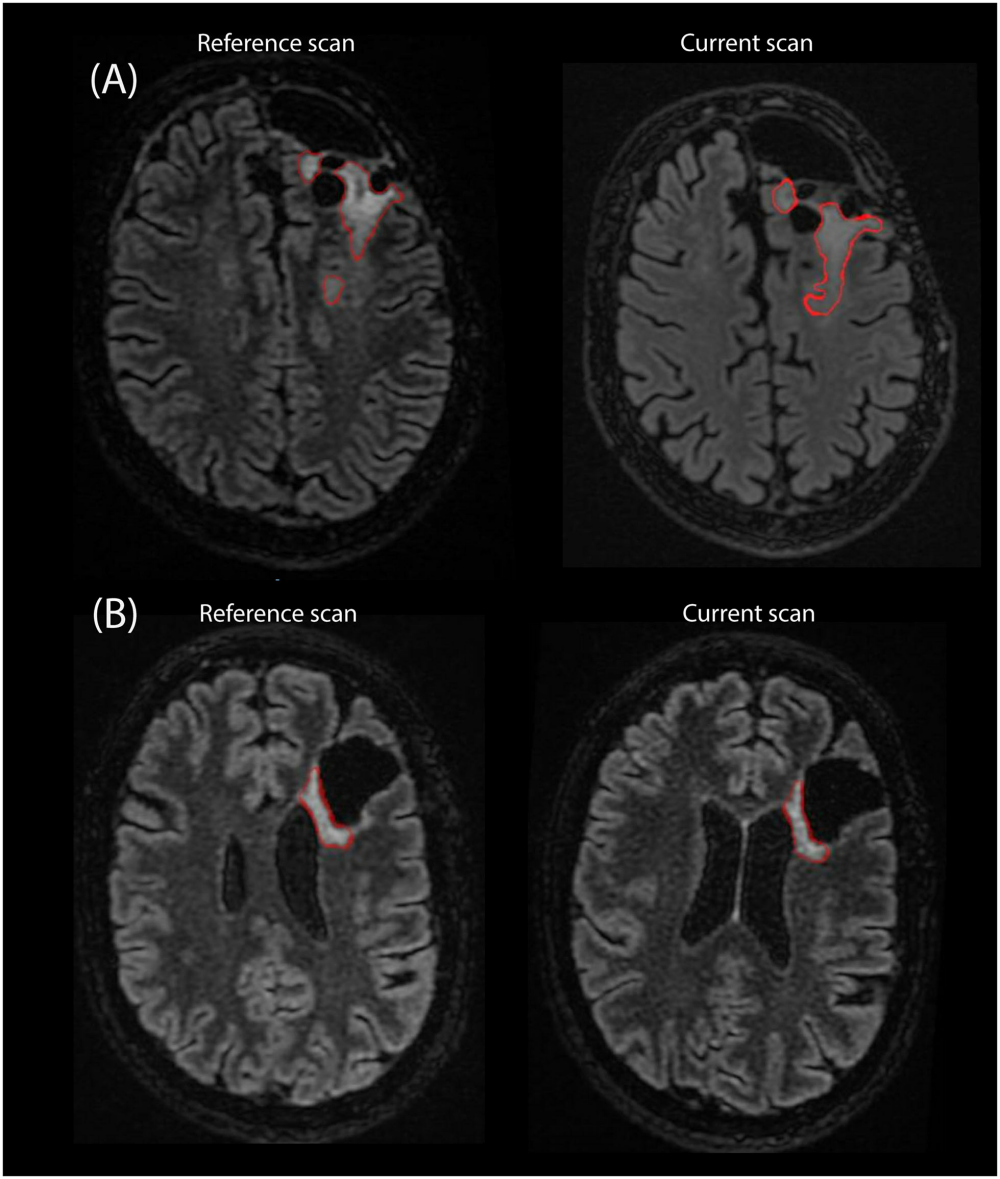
Example of segmentations as they are stored in PACS as an overlay on the T2-FLAIR scan from two consecutive timepoints. (A) Two consecutive scans of patient where EASE segmentations were considered inconsistent by the radiologist. (B) Two consecutive scans where a volume-based diagnosis of stable disease could be made.
